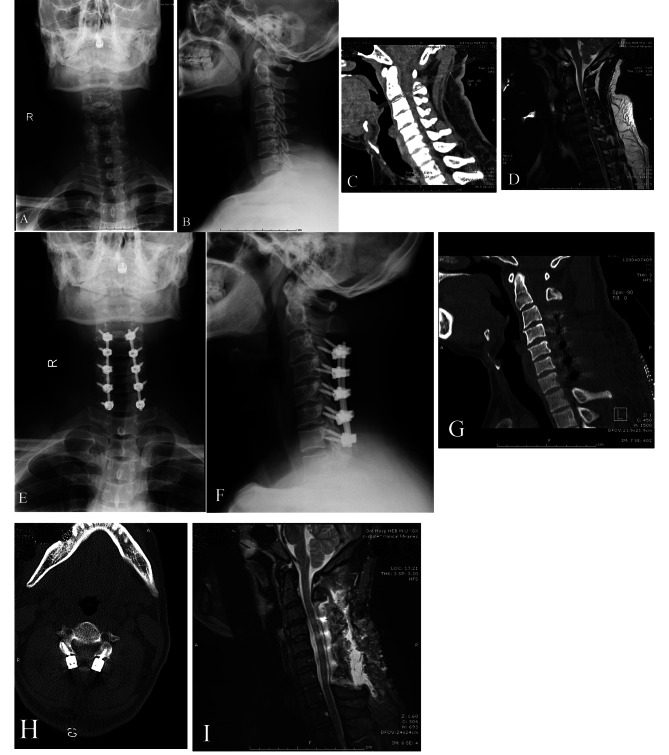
A 38 years old male patient diagnosed with MCSM underwent PCLF surgery. (A and B) Preoperative anterior and lateral X-ray radiograph of cervical vertebrae. (C and D) Preoperative CT and MRI indicate the compression from osteophyte and cervical stenosis. (E and F) Anterior and lateral X-ray radiograph of cervical vertebrae after C3-7 PCLF surgery. (G and H) Postoperative three-dimensional CT scan of cervical vertebrae indicate complicate laminectomy and accurate lateral mass screw fixation. (I) Postoperative MRI indicate a sufficient decompression of cervical spinal cord at C3-7 levels
